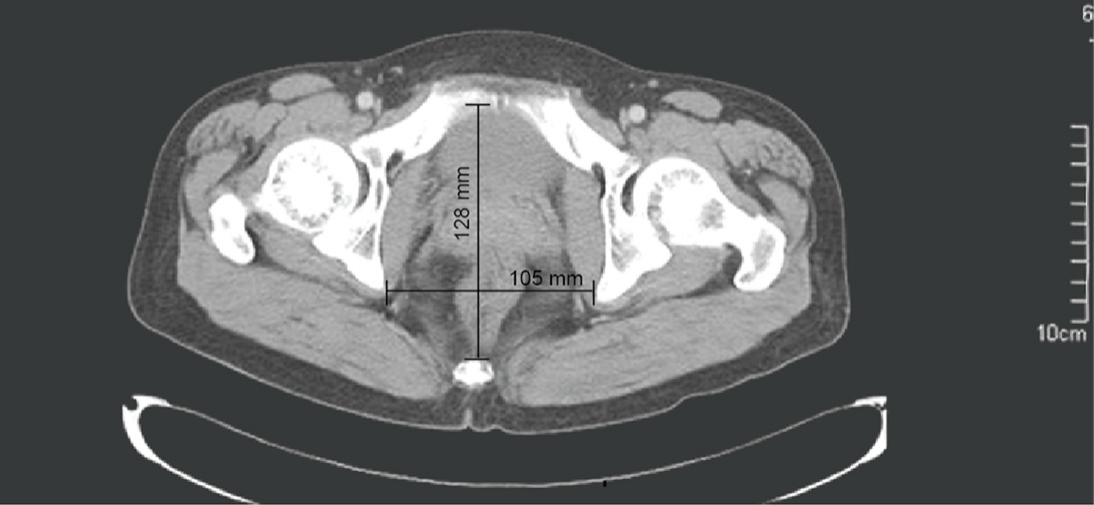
Measurements of pelvic dimensions: axial CT image showed a transverse interspinous distance of 105 mm, and anteroposterior sacrum-pubis distance of 128 mm.
